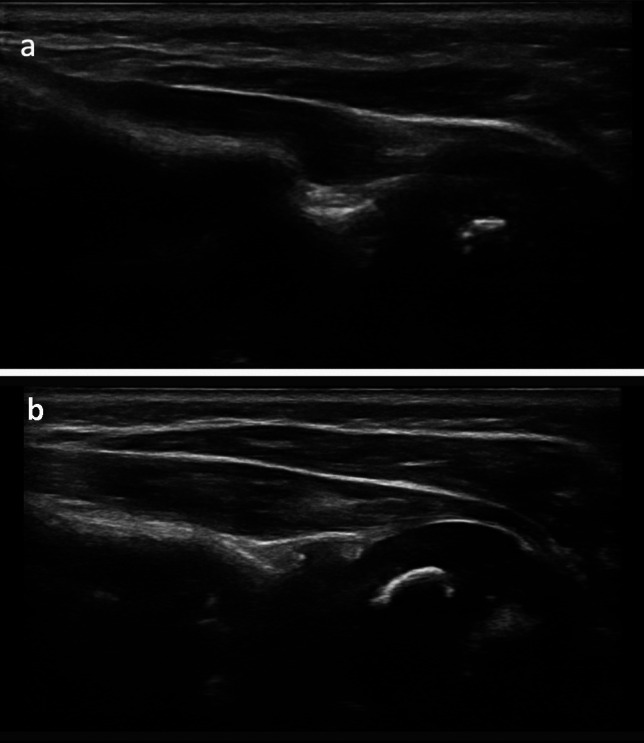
a, b Postoperative ultrasounds of two patients from the study which demonstrate an ossific nucleus anterior to the scapular line, indicating a reversal of the glenohumeral dysplasia
